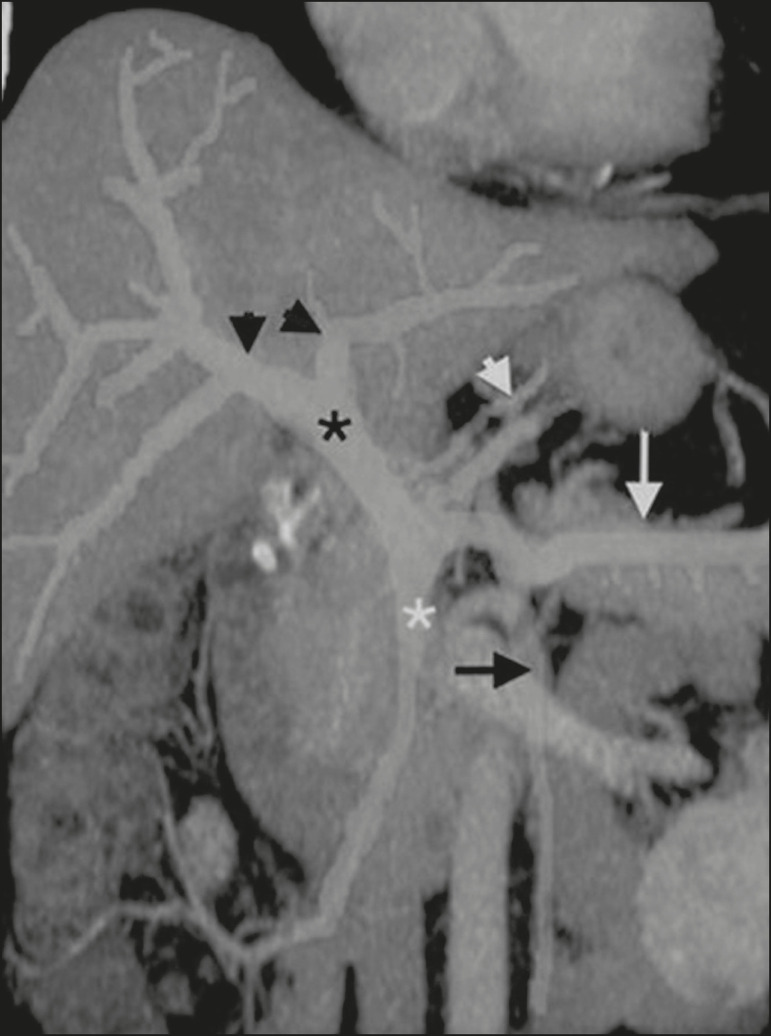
Coronal CT reconstruction showing the portal venous system. The main portal vein (black asterisk) is formed by the union of the splenic vein (white arrow) and the superior mesenteric vein (white asterisk). The right and left portal branches divide within the hepatic hilum (black arrowheads). Other tributaries include the inferior mesenteric vein (black arrow) and the gastric veins (white arrowhead).
